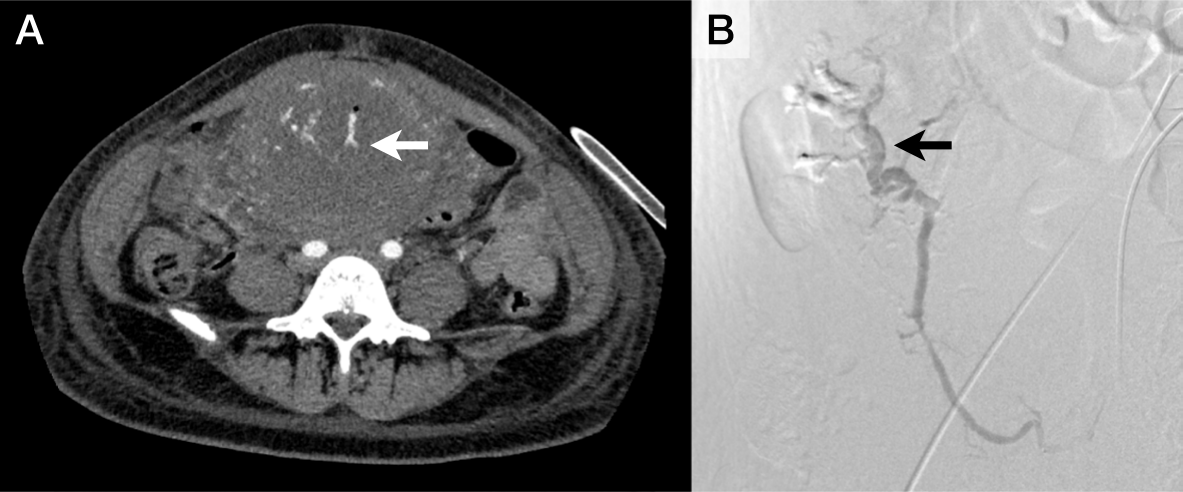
Imaging findings of arterial contrast extravasation. A Axial contrast-enhanced computed tomography at the level of the fifth lumbar vertebra, showing contrast extravasation from the right uterine artery (arrow). B Angiographic image obtained during interventional radiology, indicating contrast extravasation from a hypertrophied spiral artery at the peripheral end of the right uterine artery (arrow)
